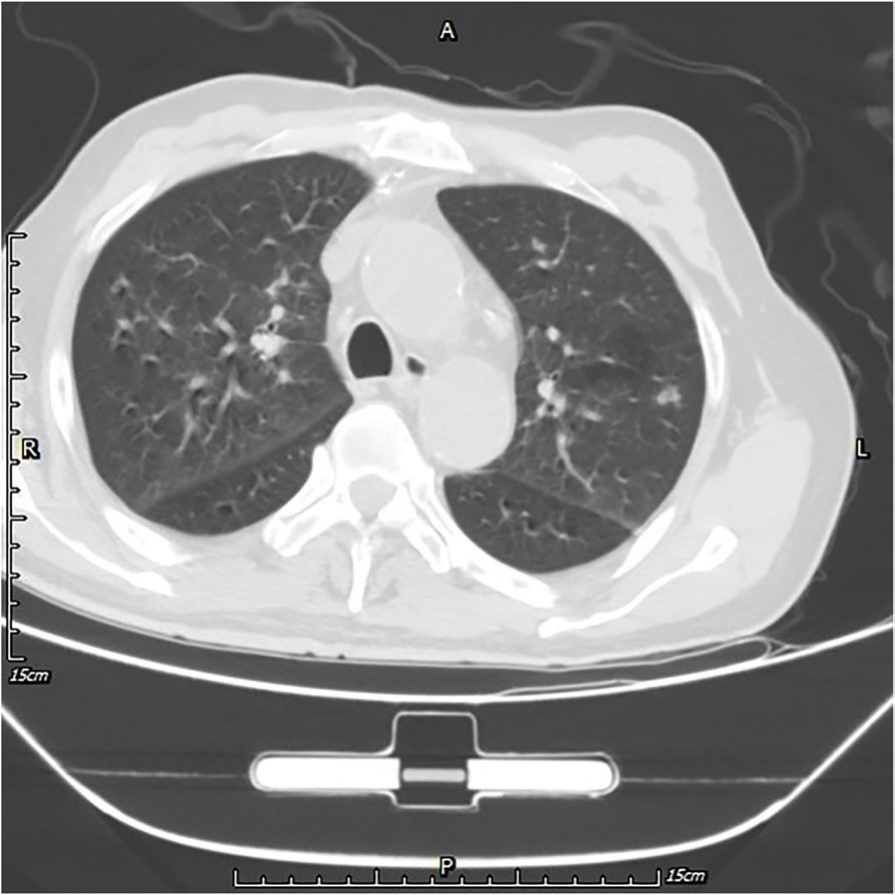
A lung CT (Computed Tomography) image showing multiple nodules in both lungs
